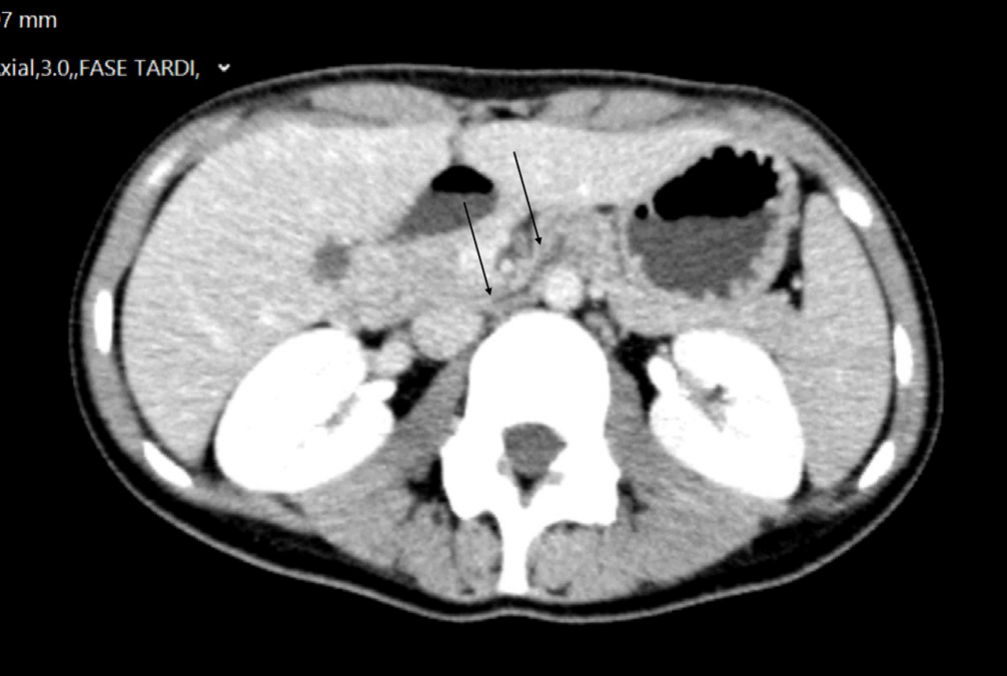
Axial plane of abdominal CT showing narrowing of the duodenum (black arrows) between the aorta and the superior mesenteric artery.
